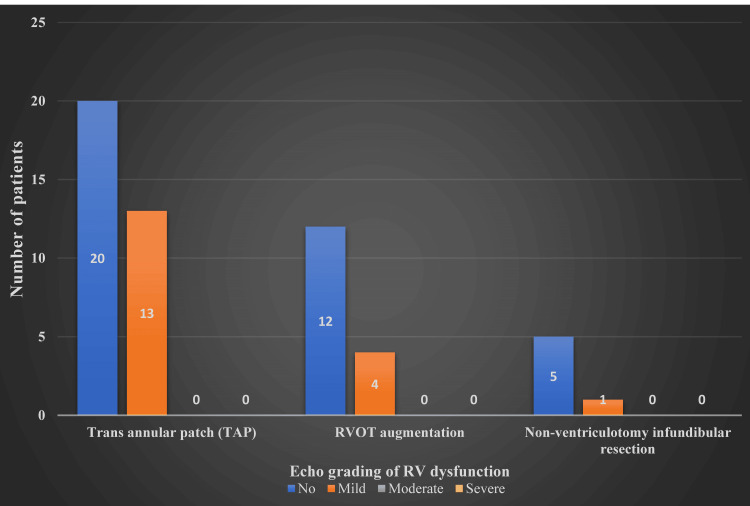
Grades of right ventricular dysfunction in follow-up echocardiography. TAP = transannular patch; RVOT augmentation = right ventricular outflow tract pericardial patch augmentation; RV = right ventricular
